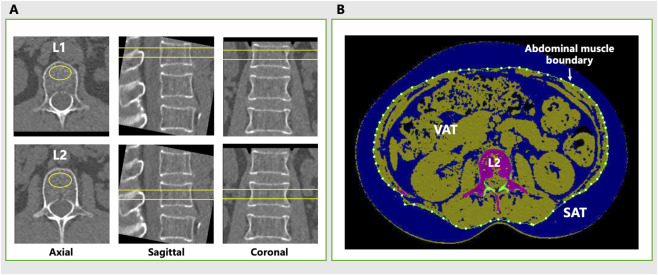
QCT-based assessment of lumbar vBMD and abdominal fat distribution. (A) Lumbar vBMD was measured by placing ROIs within the trabecular bone of the L1 and L2 vertebral bodies on axial, coronal, and sagittal images. (B) At the mid-L2 level, QCT PRO software automatically delineated the abdominal muscle boundary to differentiate SAT (between the muscle and skin) from VAT (within the abdominal cavity). Abbreviations: QCT, quantitative computed tomography; vBMD, volumetric bone mineral density; ROIs, regions of interest; SAT, subcutaneous adipose tissue; VAT, visceral adipose tissue.
